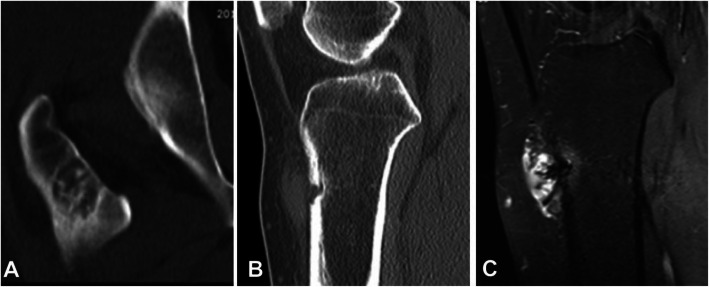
Medullary and intracortical hemangiomas. a. Coronal reconstruction of computed tomography (CT) imaging showed that the medullary hemangioma lesion was located inside the medullary cavity in the intertrochanteric femur with bone septations and osteosclerotic rims. b & c. Sagittal reconstruction of CT imaging (b) and axial T2WI magnetic resonance imaging (c) showed local wedge bone destruction with tortuous vessels in adjacent soft tissue. Enhancement scanning (b) displayed uneven enhancement in the vessels and adjacent medullary cavity
